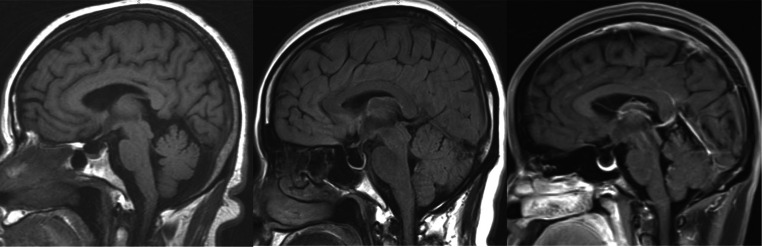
Empty sella on sagittal fat-suppressed T1WI, T2WI FLAIR and Gd-enhanced T1W1
